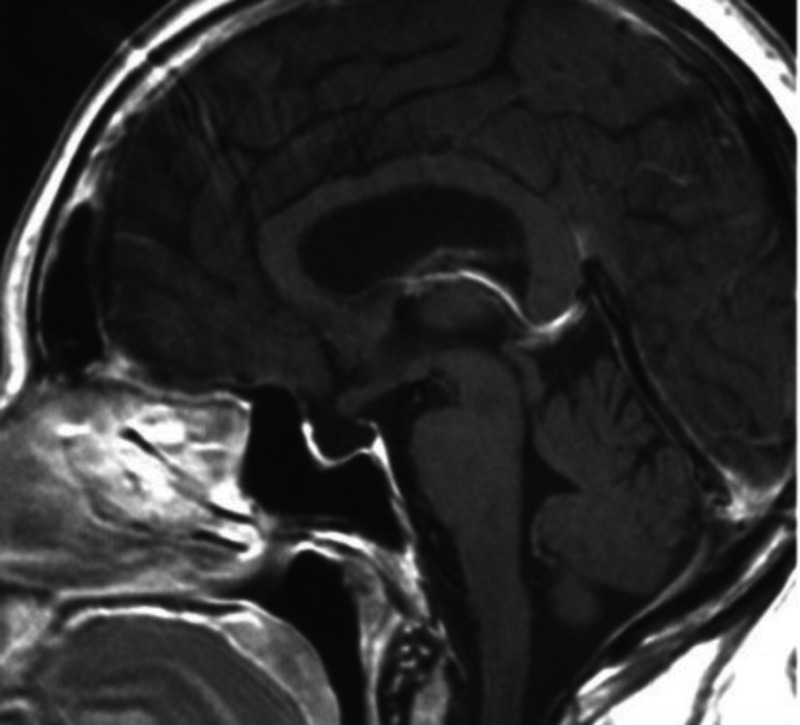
Postoperative MRI MRI: magnetic resonance imaging
